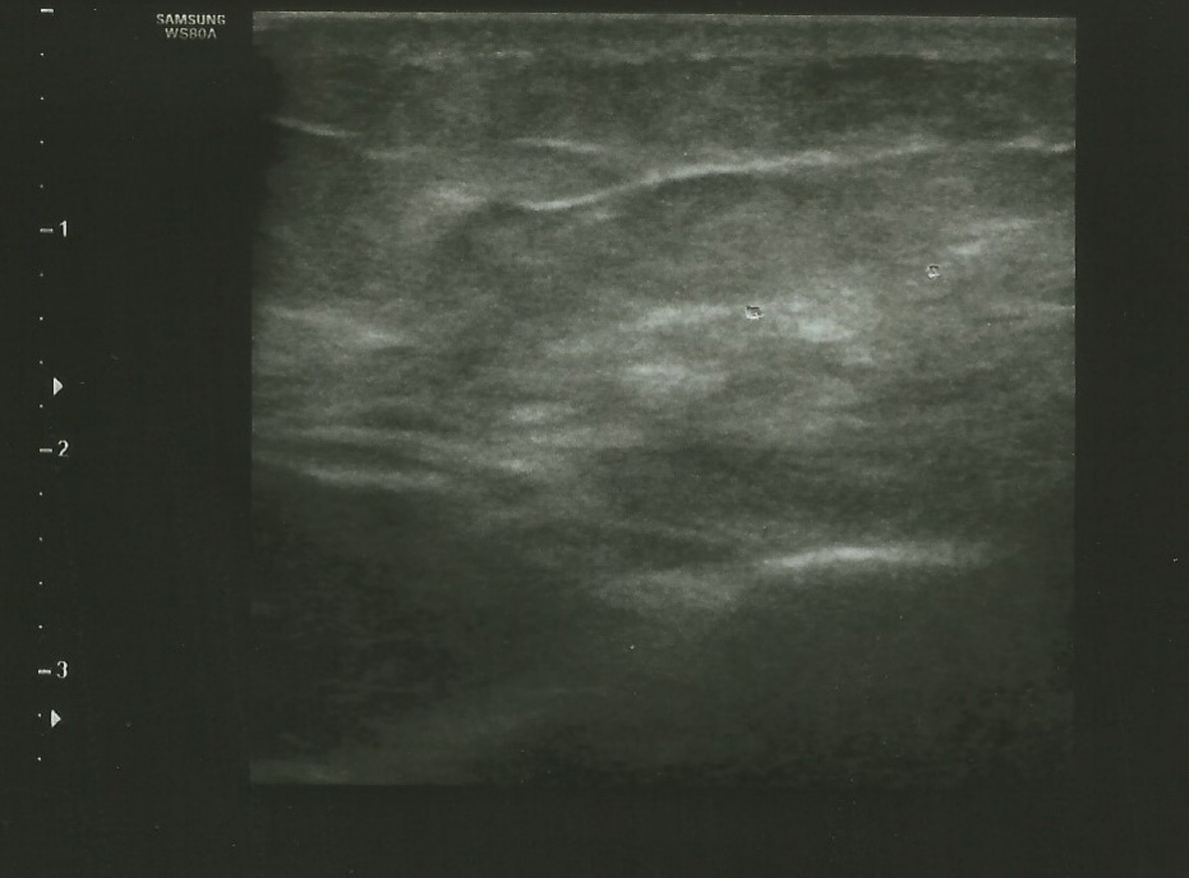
The ultra‐sonography imaging of Sputnik‐V vaccine‐induced panniculitis
